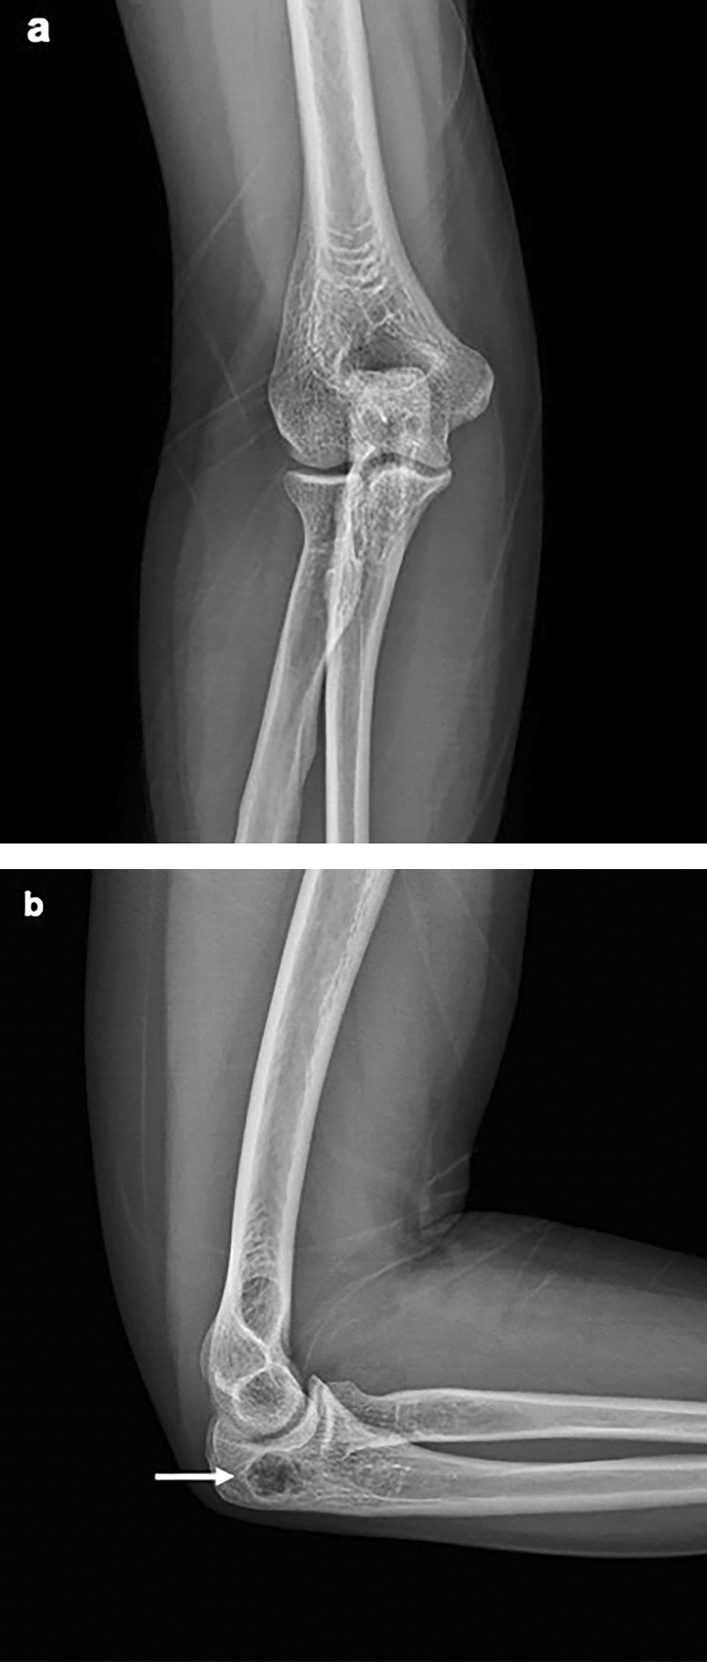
Right elbow radiograph (lateral projection). a In AP radiograph, it was difficult to find abnormality, because the olecranon process and the humerus overlapped. b A partially ill-defined osteolytic lesion ~ 2.0 cm in extent (white arrow) in the olecranon of the right ulna without discernible underlying mineralization
